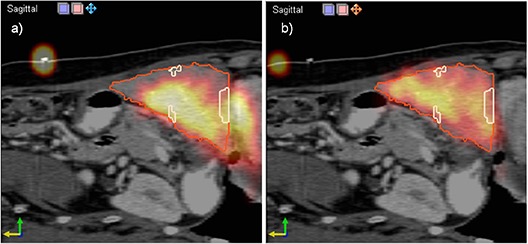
Comparison of registration methods using manual dual SPECT‐CT markers (a) and the narrow band approach (b). The largest error was observed in the manual match due to internal motion. The mutual information and narrow band registrations agree for this case.
